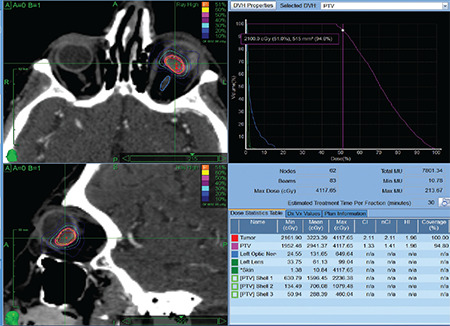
Planning Stereotactic Radiosurgery: Fusion of cranial MRI and cranial CT images of a patient with uveal melanoma in the left eye was performed using the Multiplan (Multiplan® Accuray Incorporated, Sunnyvale, California, USA) contouring software. Using the fused images, PTV was created by adding a 1-mm margin to the tumor. Non-isocentric, non-coplanar planning was done. Dose limits set for the optic nerve and lens were 7 Gy and 3 Gy, respectively. After planning, a 21-Gy treatment was performed with coverage of 100.0% of the tumor and 95.0% of the PTV. The CI was 1.31. The maximum dose to the optic nerve was 6.49 Gy and to the lens was 0.99 Gy MRI: Magnetic resonance imaging, CT: Computed tomography, CI: Conformity index, PTV: Planning target volume, Gy: Gray
